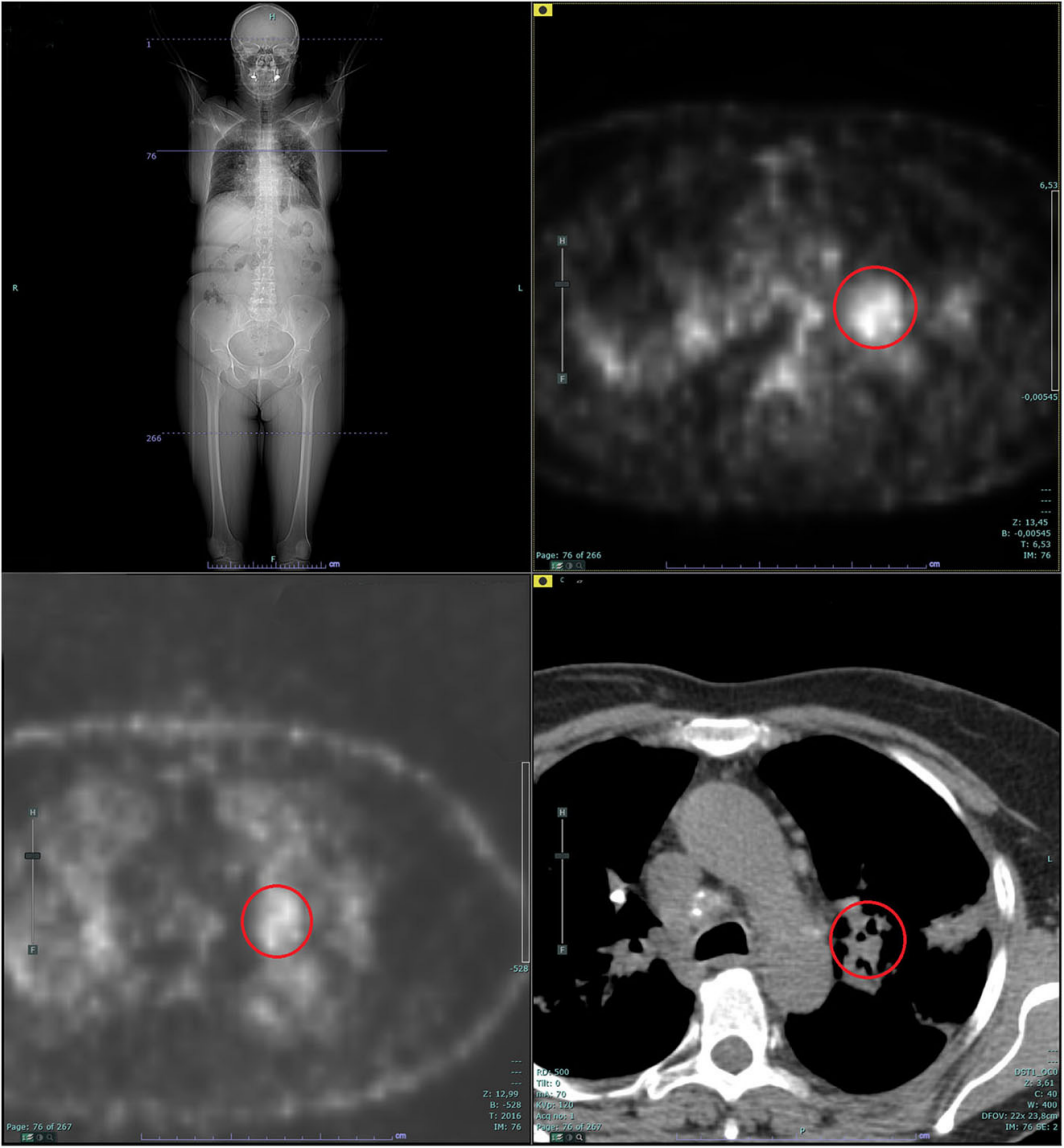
Whole-body positron emission tomography (PET): supra- and subdiaphragmatic pathologic lymphadenopathies in the absence of putative primary mass detection. The red circle shows the pathologic standardized uptake value (SUV) at left hilar lymph node (L10 station).
